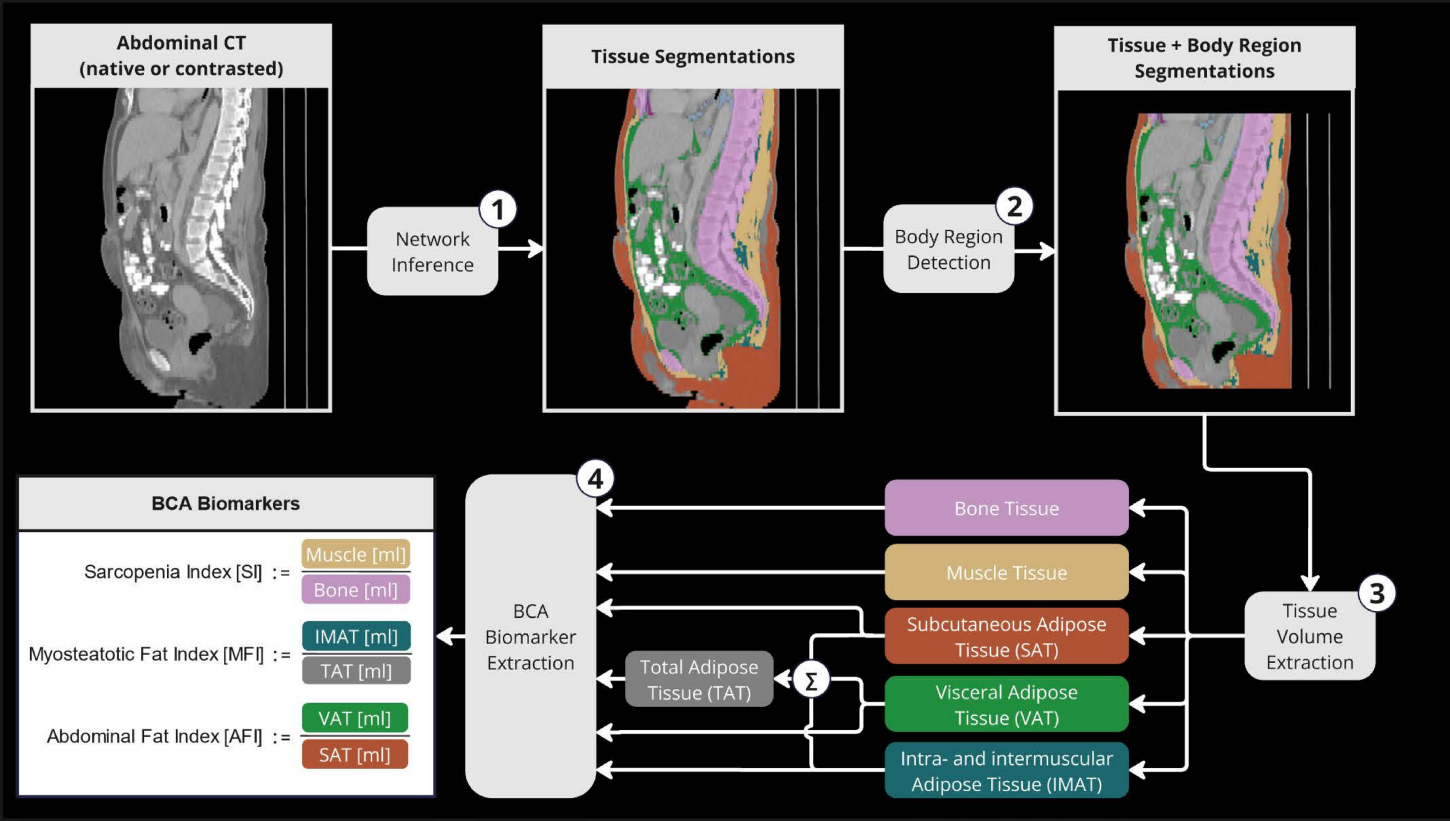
Overview of the body composition analysis (BCA) inference and marker extraction. Routine CT abdominal or whole‐body scans were introduced as input to the network. Muscle, bone and multiple adipose tissues were extracted from a uniform body region (abdominal cavity) in millilitres. In a final step, the extracted volumes were combined into specific markers: Sarcopenia Index (SI), Myosteatotic Fat Index (MFI) and Abdominal Fat Index (AFI).
